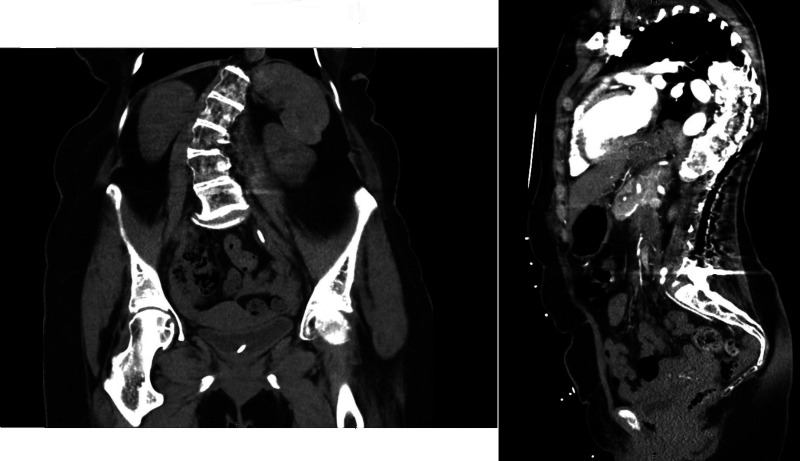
Computed tomography (CT) of the abdomen and pelvis with and without i.v. contrast of patient showing severe S-shaped scoliosis (*left*) and CT angiography (CTA) of the chest showing kyphoscoliosis.
